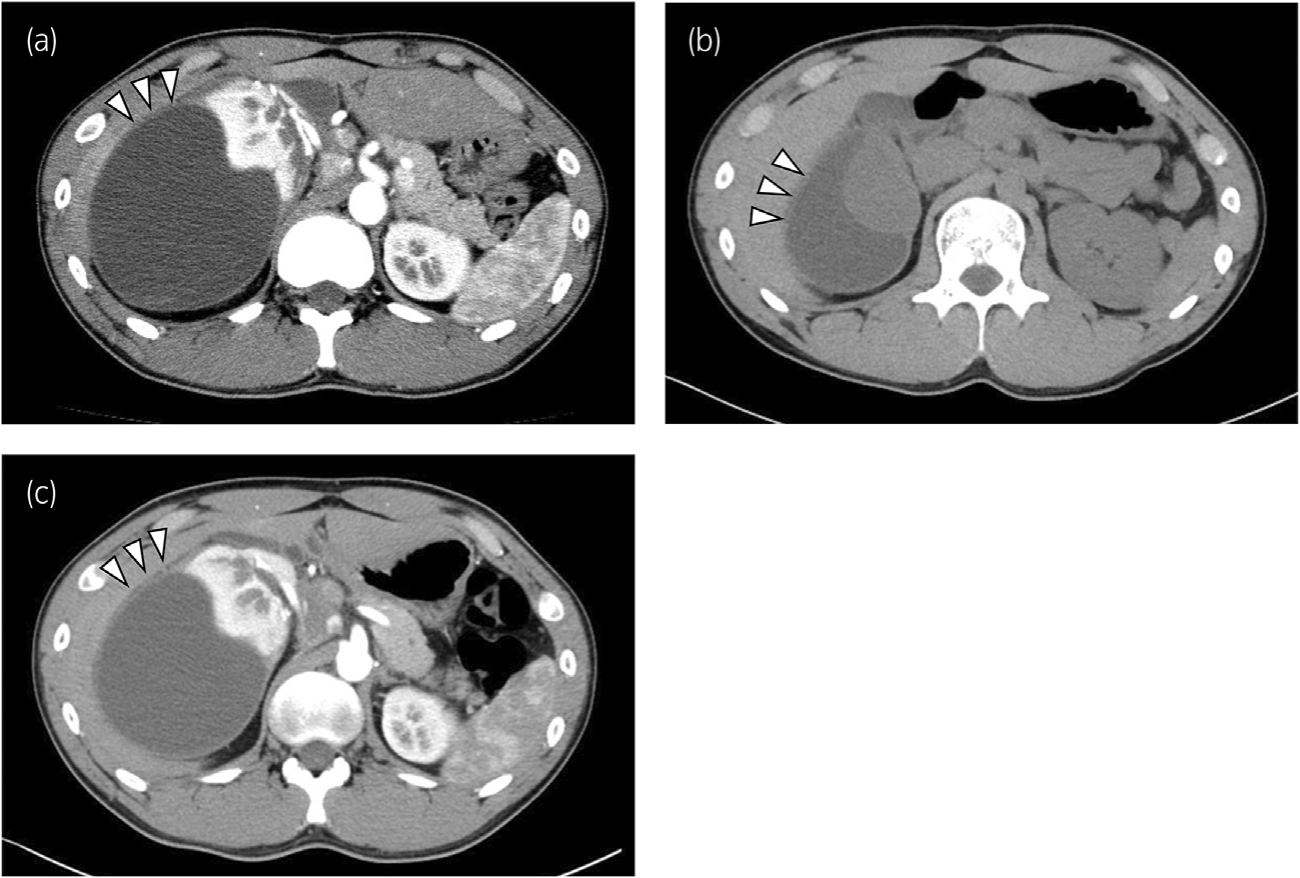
Fig. 1a,c are contrast CT images. Fig. 1b is a plain CT image. (a) CT showed a large encapsulated cystic lesion (measuring approximately 14 × 10 × 12 cm) around the right kidney (white arrowheads), with severe external compression of the renal parenchyma. (b) One month after of percutaneous drainage of the right perirenal hematoma, CT showed volume reduction of the hematoma (white arrowheads) and improvement of external compression of the renal parenchyma. (c) Six months after percutaneous drainage, the right perirenal hematoma enlarged again (white arrowheads).
